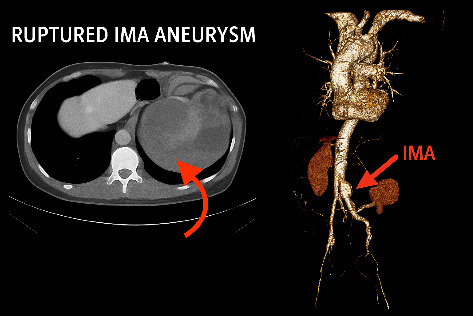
Axial CT and 3D CTA images demonstrating ruptured IMA aneurysm and associated aortic dissection (red arrows).
